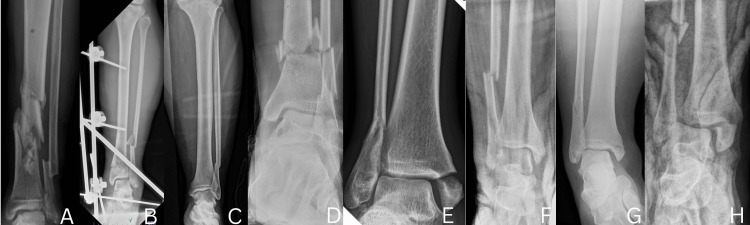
Preoperative radiographs of subjects with various types of ankle fractures treated using fibular nail fixation This figure is original and derived from data collected in the present study. The alphabetical identifiers (e.g., A, B, C, etc.) used in the figure are arbitrary and were created solely for the purpose of referencing specific cases within this article. These identifiers do not correspond to any patient-identifying information.
